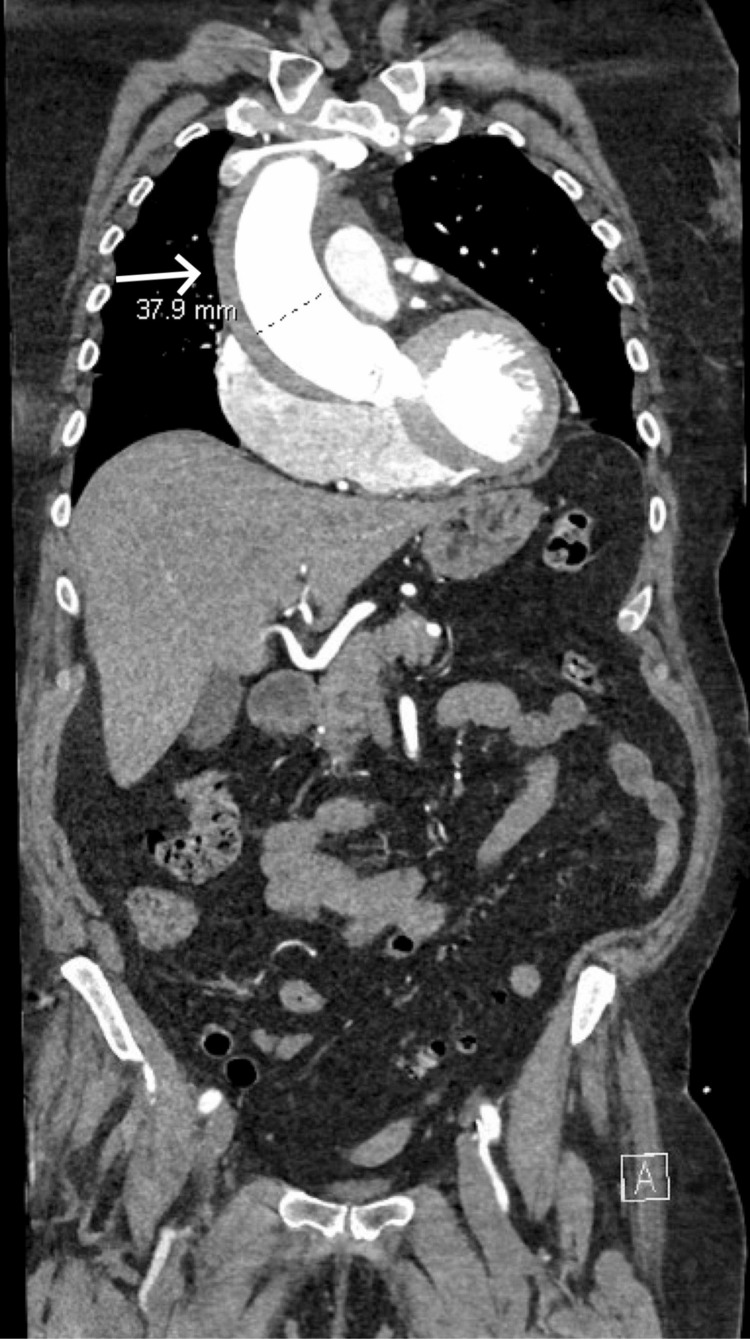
Coronal computed tomography angiography (CTA) demonstrating type A aortic intramural hematoma. Coronal CTA shows crescentic, hyperattenuating thickening of the ascending aortic wall (arrow), measuring approximately 37.9 mm, consistent with intramural hematoma. The abnormality extends from the sinotubular junction into the descending thoracic aorta. Notably, there is no visible intimal flap or double lumen, distinguishing this from classic aortic dissection.
